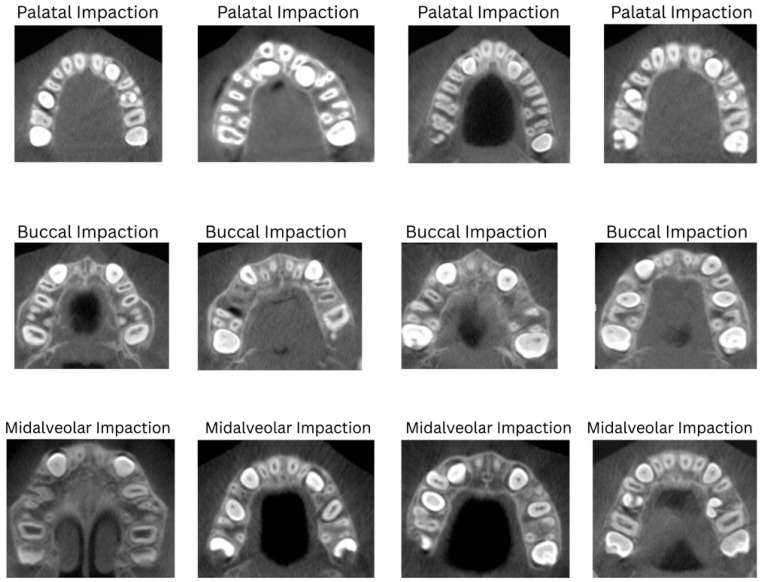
CBCT for impaction spatial information sample set.
